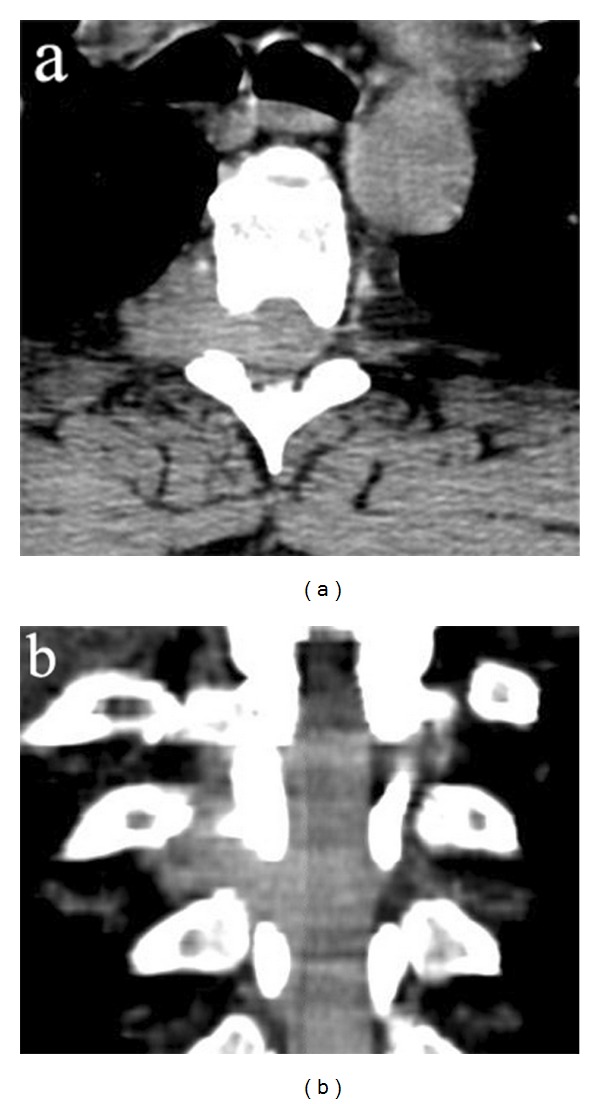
Preoperative axial (a) and coronal (b) computed tomography scans. Computed tomography scans revealing a dumbbell-shaped intra-extra-spinal mass at the D4–D6 level.
